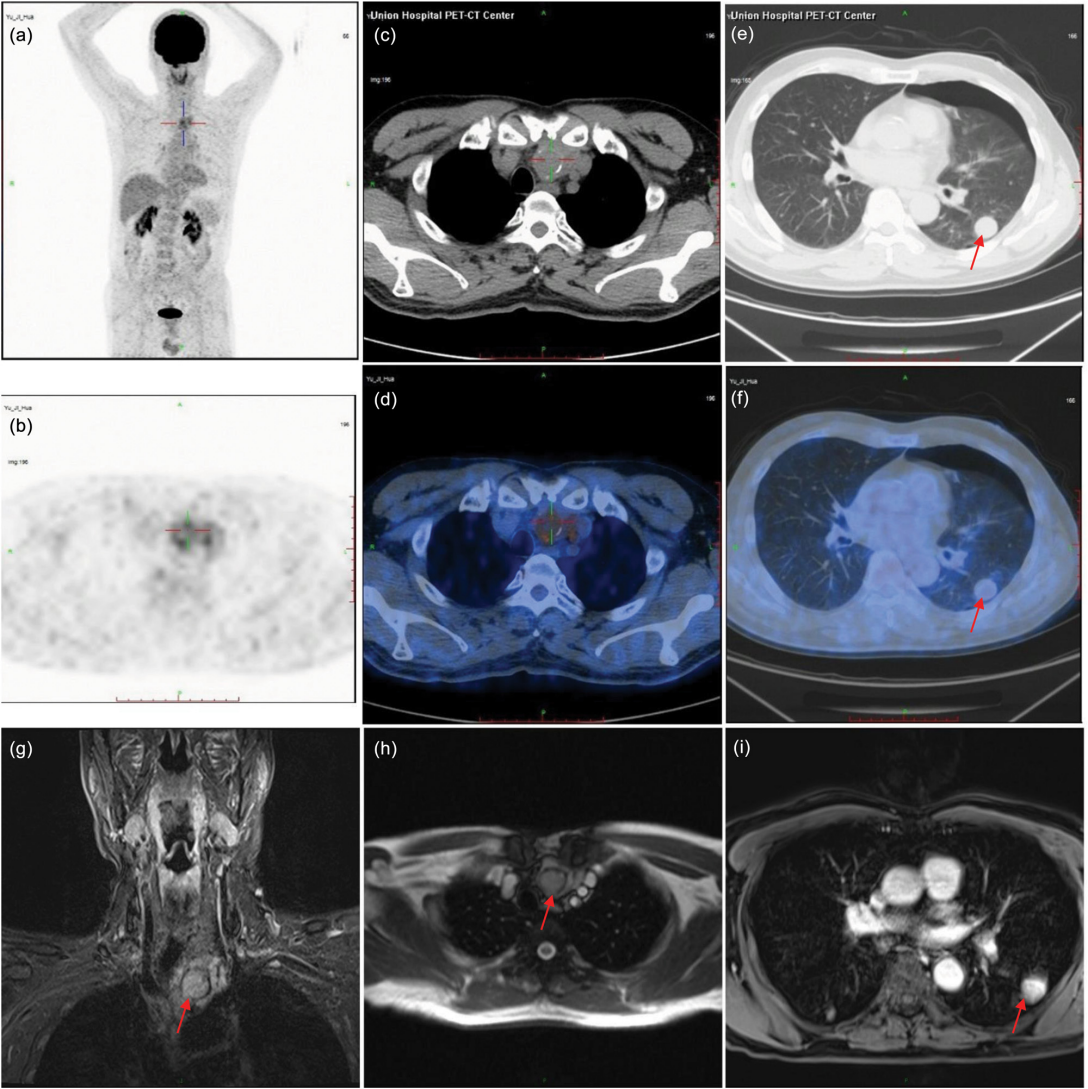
Imaging findings of the patient. (a–d) PET/CT showed a mass in the mediastinal portion. (e and f) PET/CT showed nodules (red arrow) in lung. (g and h) MRI examination showed a 4.5 cm × 3.0 cm × 3.8 cm mass (red arrow) in the mediastinal portion. (i) MRI examination showed multiple nodules in the lung. The red arrow indicated a 1.9 cm × 1.8 cm nodule in the left lower lobe of the lung.
